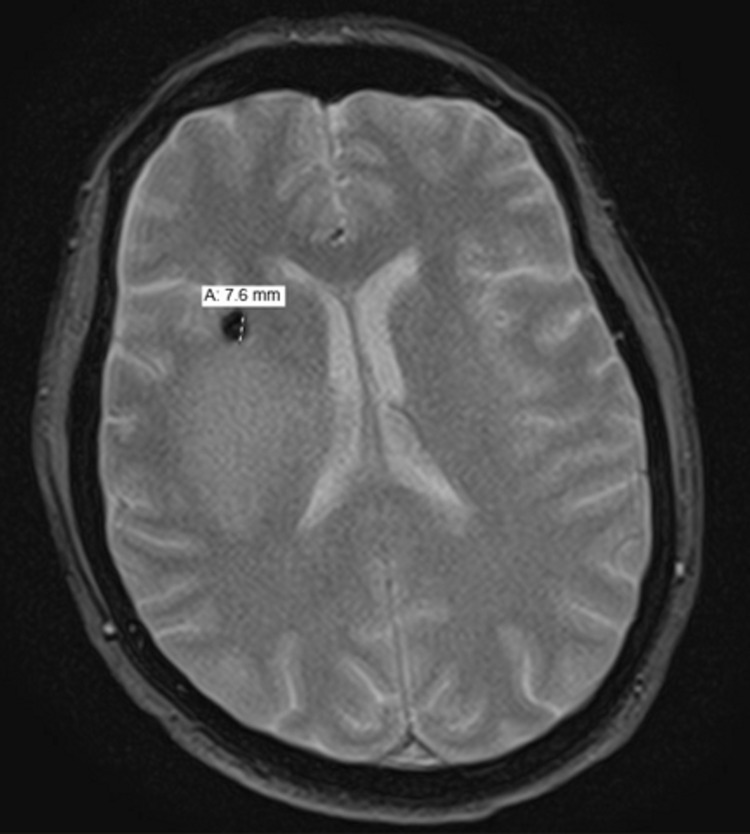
Pre-op MRI showing mass with surrounding vasogenic edema.
